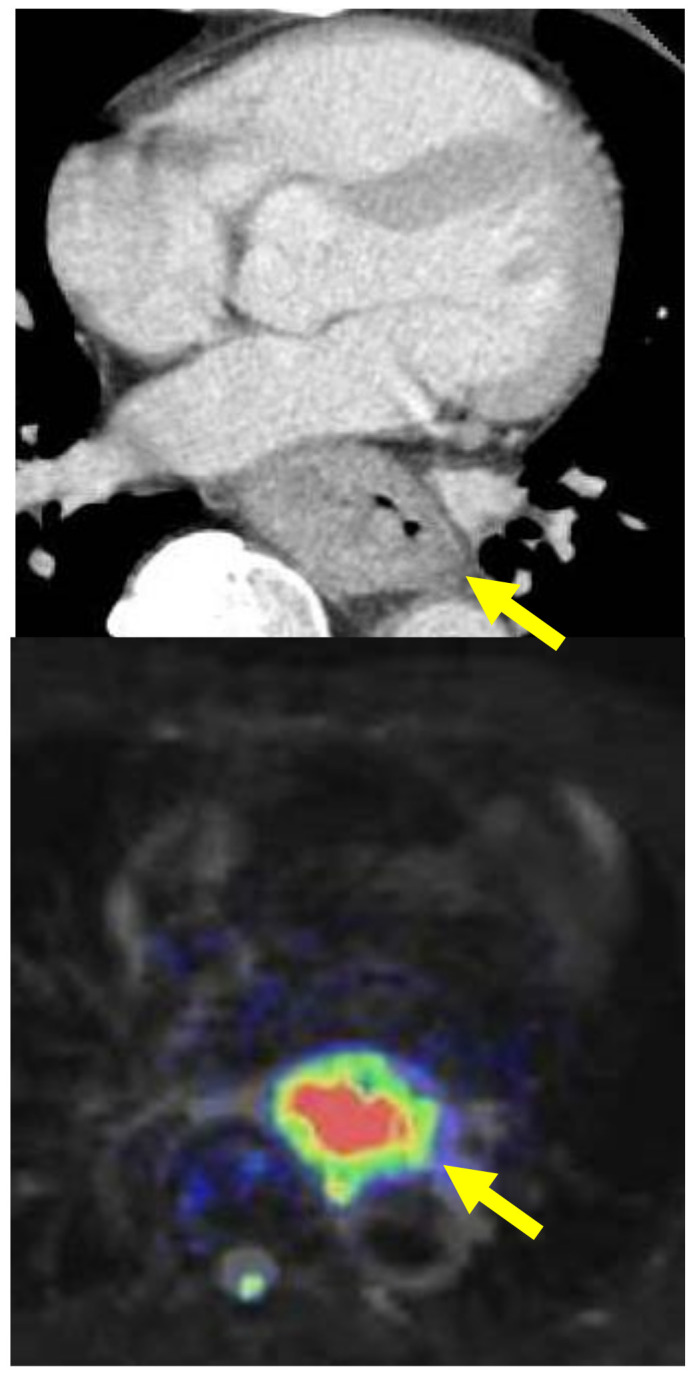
(Upper) Contrast-enhanced CT image. (Lower) Diffusion image obtained with 1.5T-MRI for esophageal squamous cell carcinoma. Arrows indicate the esophageal tumor.
